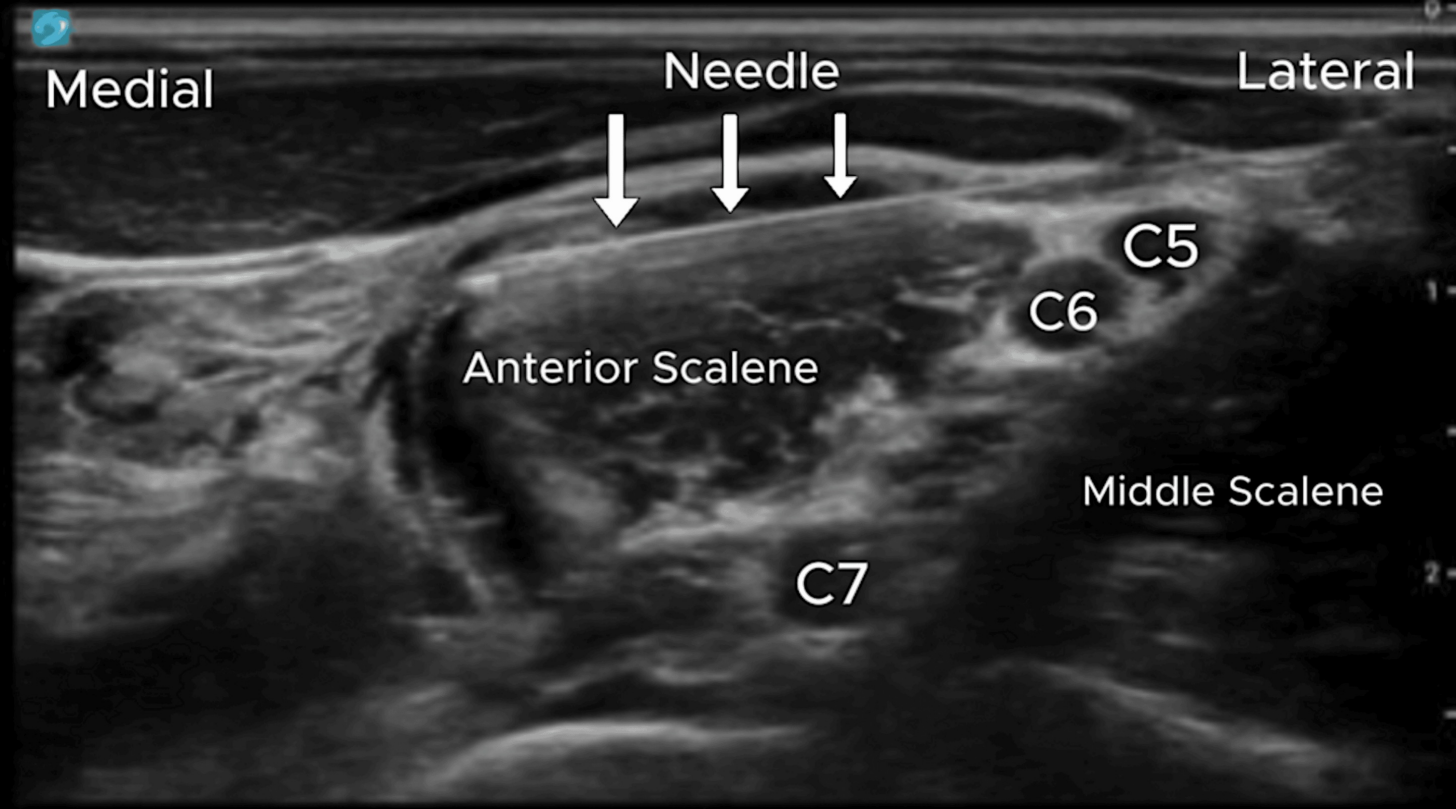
Ultrasound image demonstrating right phrenic nerve block (PhNB). The image labels anatomical landmarks, including the anterior and middle scalene muscles, cervical vertebrae (C5, C6, C7), and needle trajectory (white arrows). The phrenic nerve is typically located anterior to the anterior scalene muscle. This image is original and was obtained from one of the cases included in this study.
